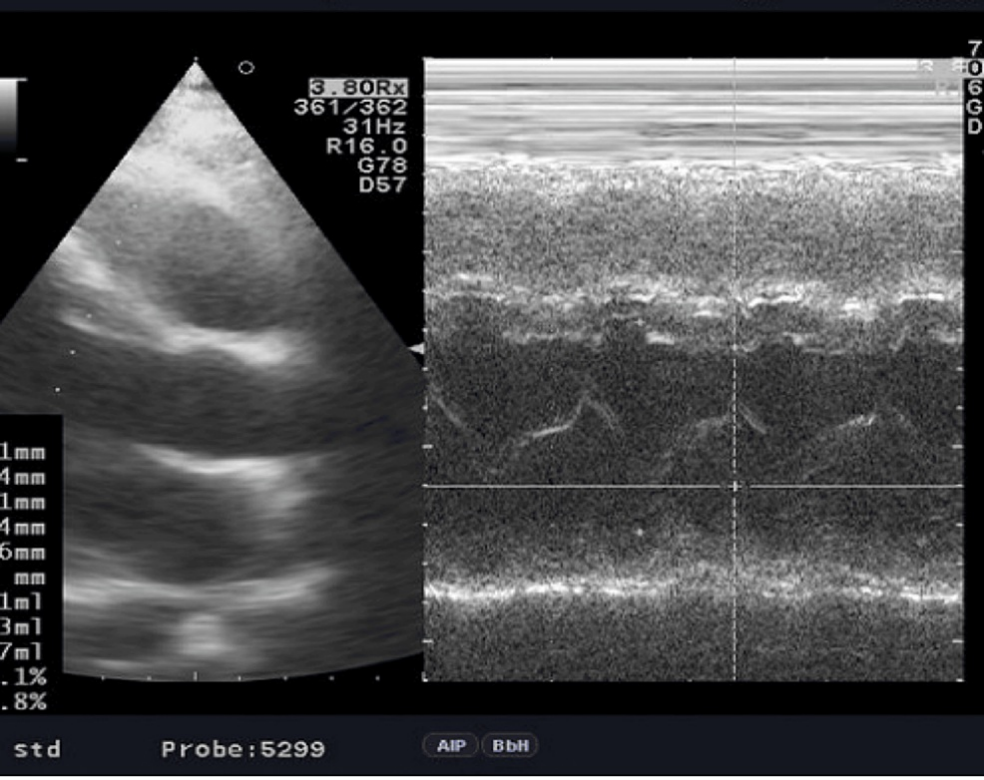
A transthoracic echocardiogram (TTE) of the first sibling showed apical hypokinesis.
